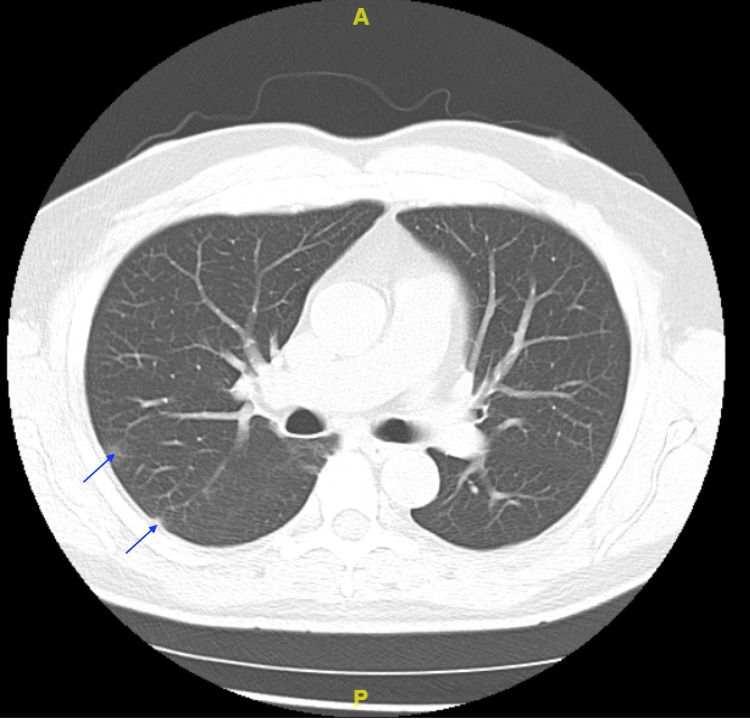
CT scan of the chest (axial view) demonstrating subtle ground-glass opacities (arrows)
